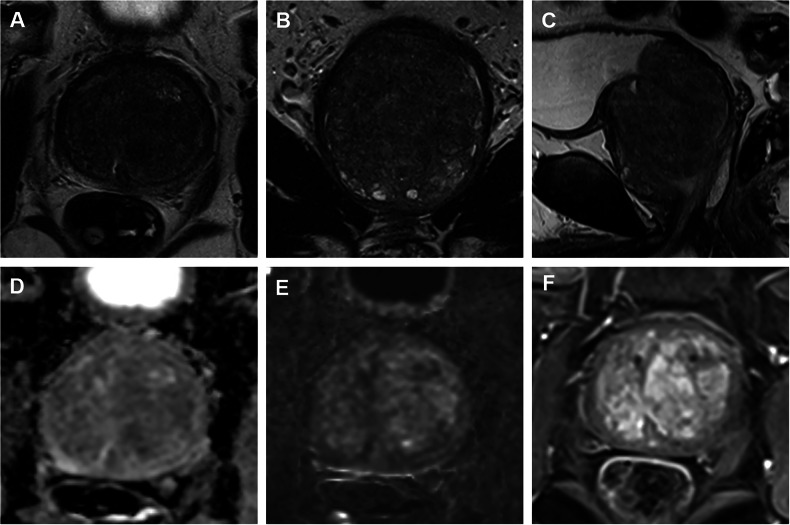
Sixty-five-year-old man with a PSA value of 24 ng/mL. Diffuse changes on T2 images with severe hyperplasia (A–C), no DWI disorder (D, E), and no focal or asymmetric contrast enhancement on DCE (F). PI-RADS classification 2. Negative at SB
